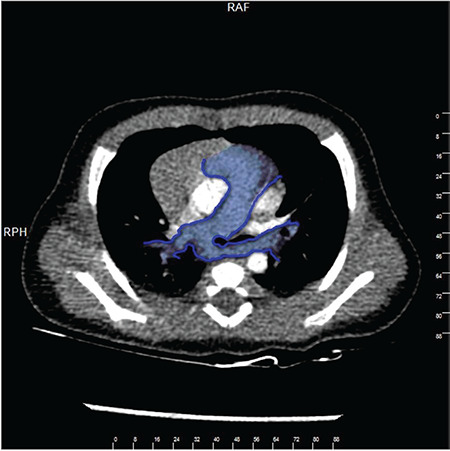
Axial contrast-enhanced computed tomography confirmed the aberrant LPA LPA: Left pulmonary artery
